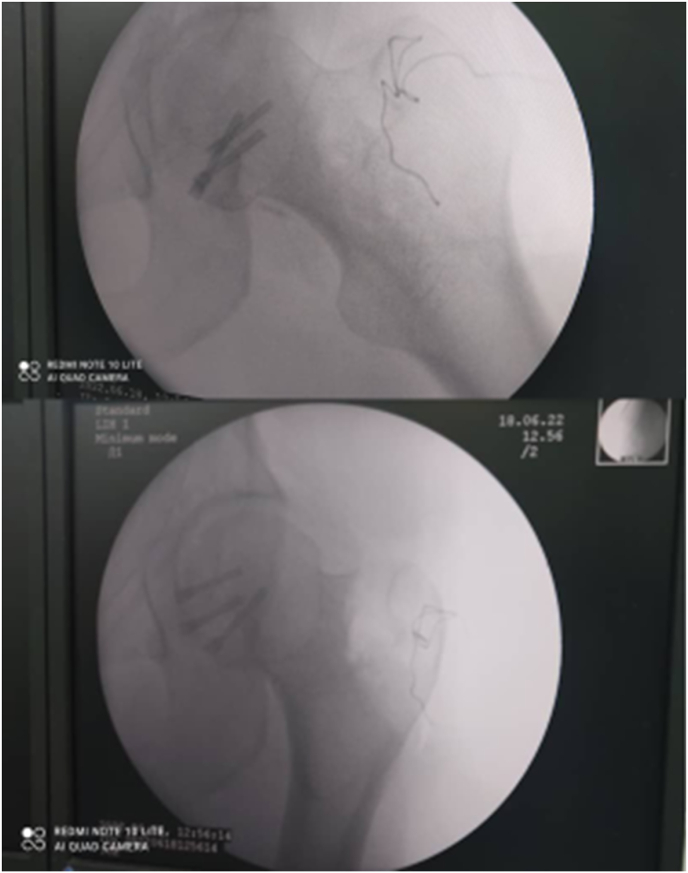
Postoperative pelvic X-ray showing screw fixation of the fractured fragment of the femoral head using three Herbert Screws. It is showing a good anatomical reduction.
